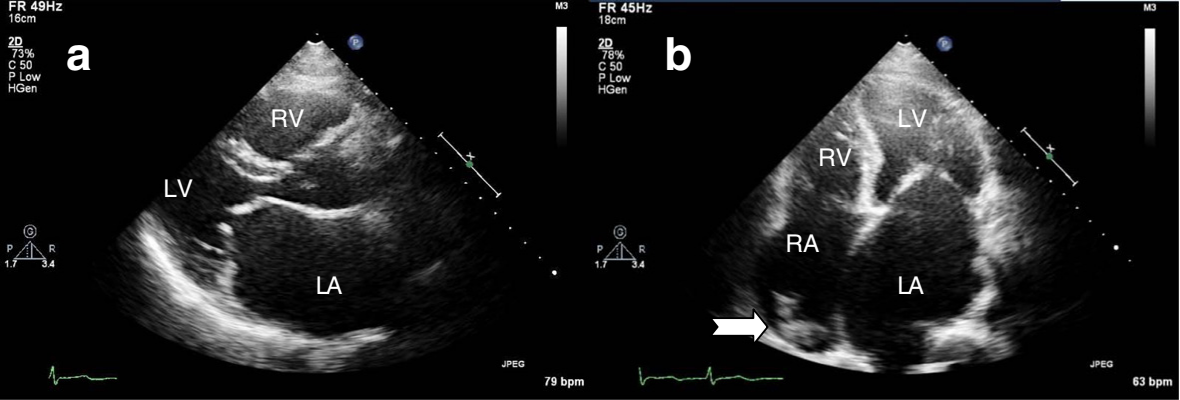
Outcome after long-term anticoagulation therapy. The left atrial thrombus is completely resolved as viewed from parasternal long axis (a) and apical four chamber view (b) in the left atrium. A residual right atrial thrombus (*notched arrow*) is still visible in the right atrium (b). *LA* left atrium, *LV* left ventricle, *RA* right atrium, *RV* right ventricle
